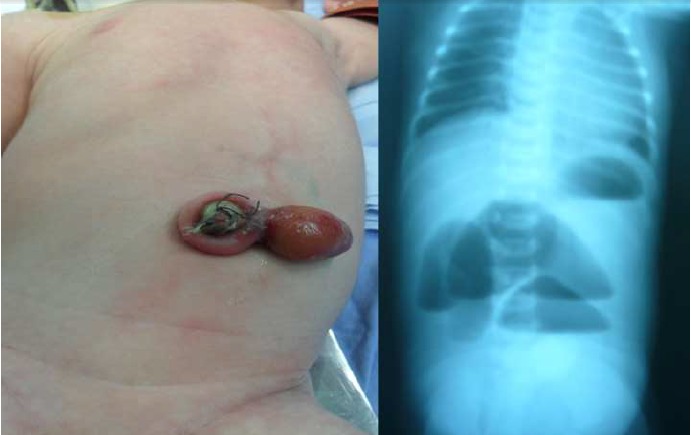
Figure 1: Extra-abdominal bowel remnant and x-ray showing small bowel obstruction.
